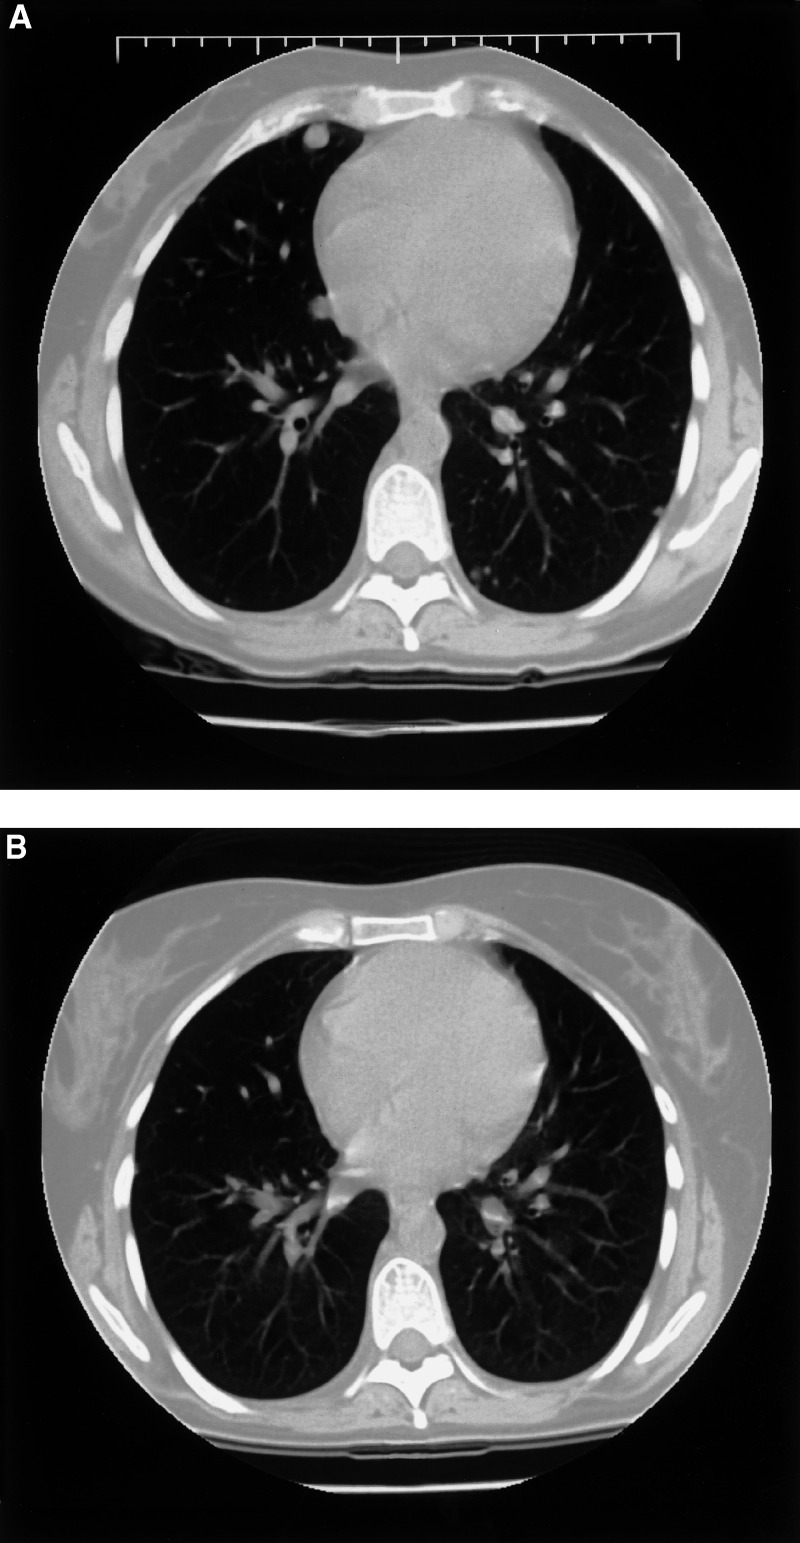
Axial CT section after three courses of chemotherapy (A) reveals multiple nodules of variable size consistent with metastases with a predominantly subpleural localisation. After IFN alpha-2b therapy, almost complete disappearance of the multiple subpleural metastases is shown (B).
